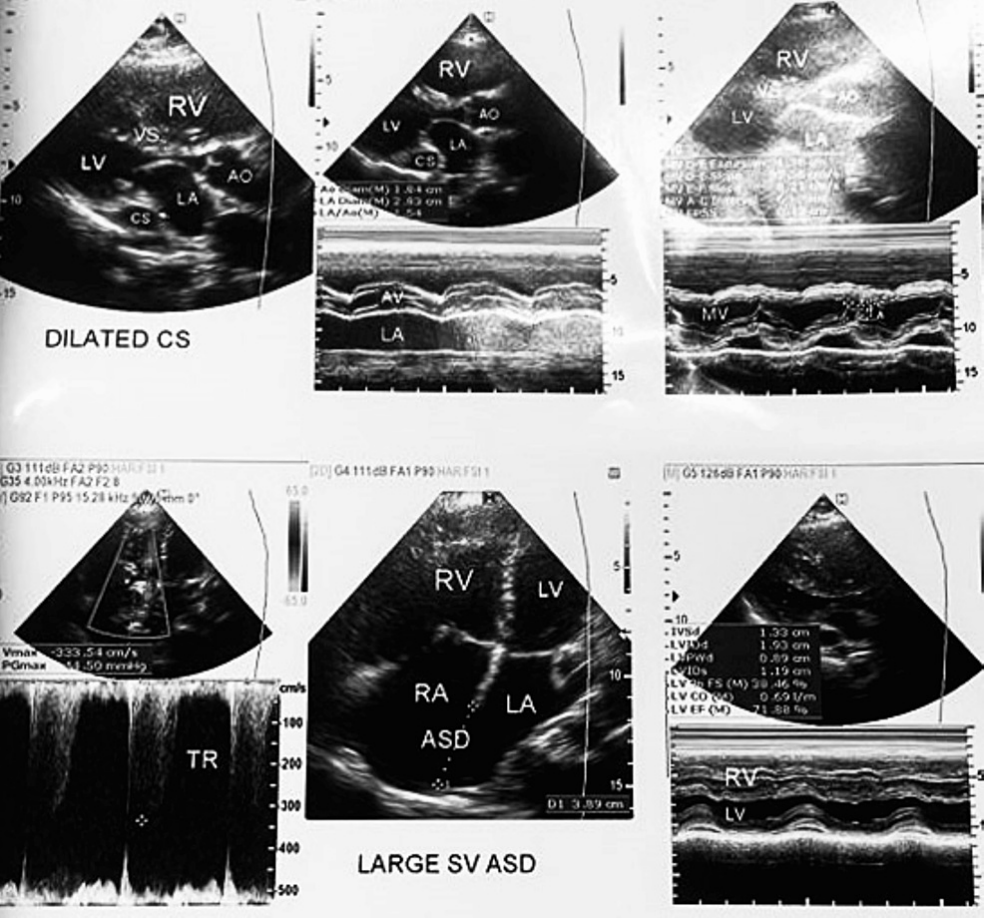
The transthoracic echocardiogram showing large sinus venosus atrial septal defect (superior vena cava type 40 mm), non-restrictive left-to-right arterial shunt, and pulmonary to systemic flow ratio (Qp/Qs) > 2.5:1 with marked right-side volume overload and preserved biventricular function
